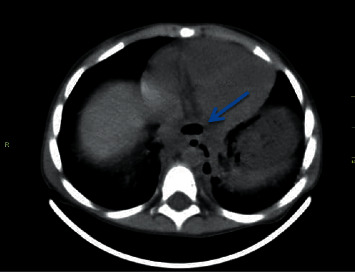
A CT scan showed the esophagus and air bubbles which can be from ruptured esophagus. The esophagus is dilated with thickened walls. It is difficult to see loculated pleural effusion and pulmonary abscess.
